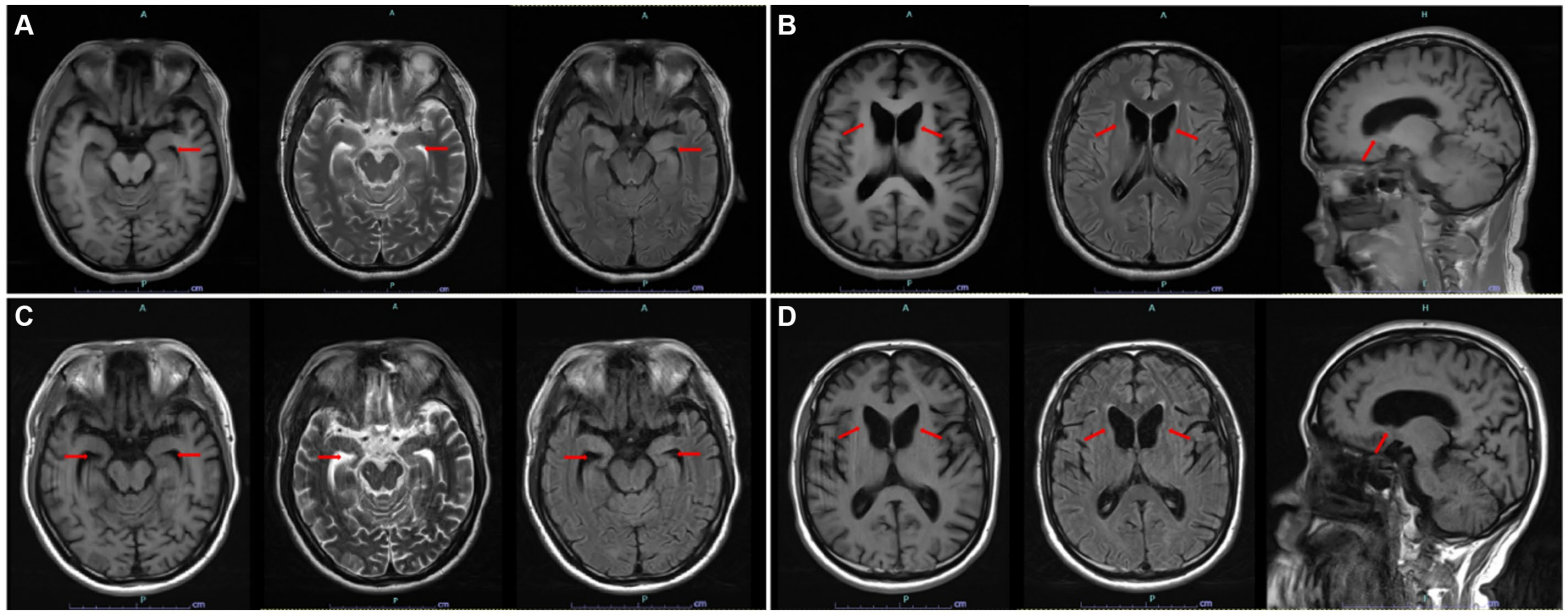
(A) Swollen left hippocampus and parahippocampal gyrus on axial T1, T2, and T2 FLAIR sequences and hyperintensity on T2 FLAIR sequences. (B) Atrophy of the head of the caudate nucleus and resulting enlarged anterior horn of the lateral ventricle on axial T1, T2Flair, and sagittal T1 sequences. (C) Decreased volume of the bilateral hippocampus and enlarged bilateral temporal horn of the lateral ventricles. (D) Almost the same degree of atrophy of the head of the caudate nucleus compared with last year. [(A,B) First structural brain MRI. (C,D) Reexamined structural brain MRI 1 year later. All images are marked by red arrows].
